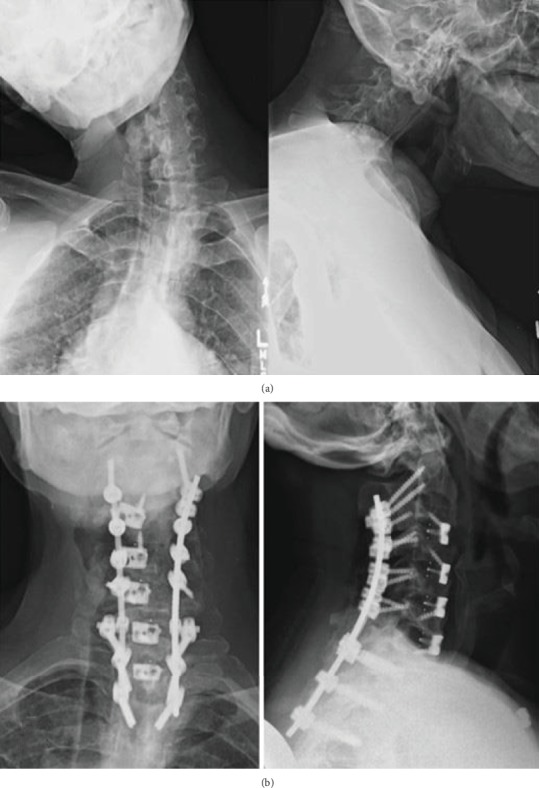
(a) CD patient's preoperative cervical X-rays demonstrating a significant coronal deformity (C2-T2 Cobb angle = 50.2°) with poor sagittal alignment (C2-7 SVA = 93.5 mm); (b) the patient's postoperative cervical X-rays demonstrating correction of fixed coronal deformity and improvement in sagittal alignment (cited from Tan and Riew [43]).
